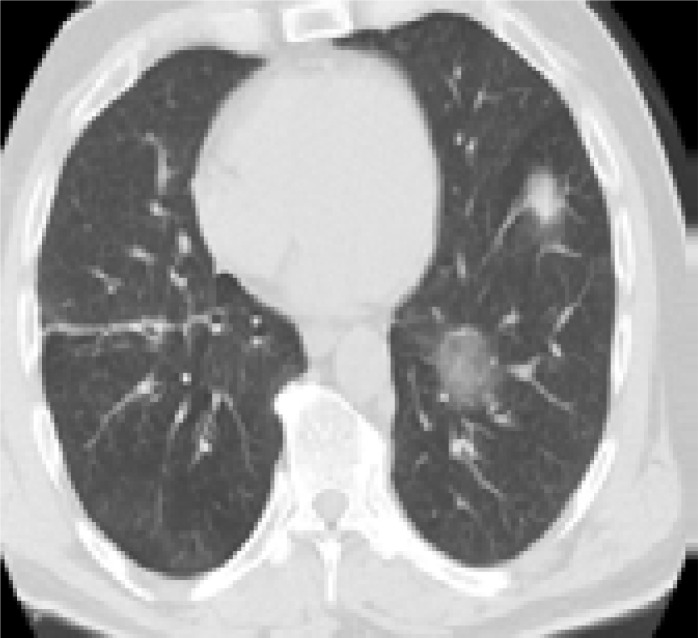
F) An 87-year old female patient with CAD+DM+HT history applied to hospital with complaints of cough and dyspnea for 1 week. Dispersed focal and reticular ground glass densities, more specifically in peripheral segments, in the parenchyma of both lungs were found in chest CT of the patient (black oval)
